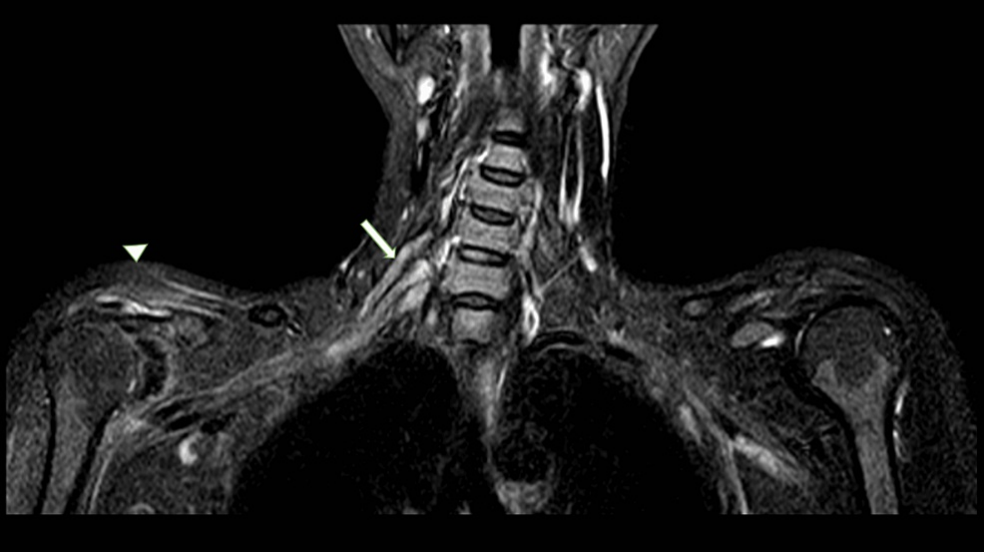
MRI-brachial plexus STIR coronal section of case 3 MRI: Magnetic resonance imaging short tau inversion recovery MRI of the brachial plexus using the STIR coronal section showing edematous the superior, middle, and inferior trunks situated within the interscalene triangle, as indicated by a large white arrow. Additionally, the shoulder muscles (comprising the supraspinatus, infraspinatus) show mild atrophic changes accompanied by fatty infiltration, highlighted by small thick white arrowheads (top of form).
